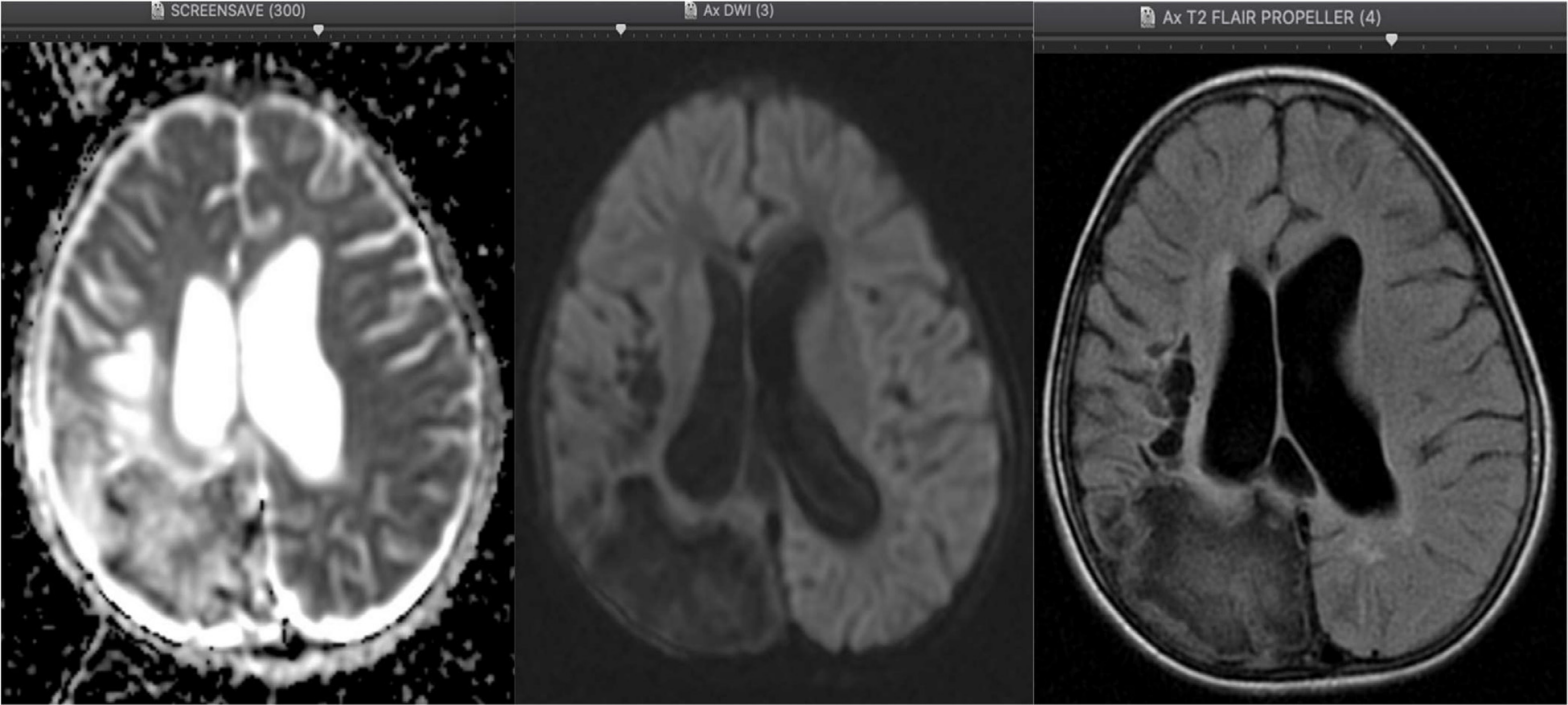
The axial section of the DWI (diffusion-weighted imaging), there is no restricted diffusion area on DWI, no suppression of lesion, and perifocal edema on axial section of the T2 FLAIR (Fluid-attenuated inversion recovery) MRI.
